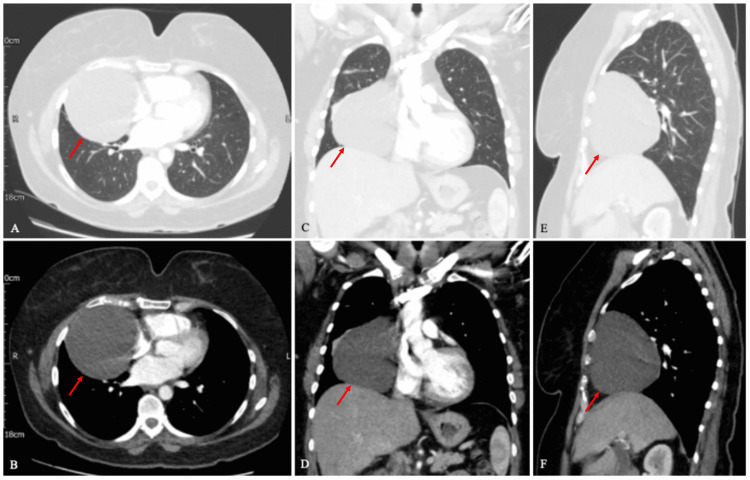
A computed tomography (CT) scan of the chest showing a large, homogenous, well-circumscribed lesion in the right lower hemithorax, and abutting the right cardiac border (red arrow). (A, C, E) Lung window (demonstrating the lung parenchyma in detail, including the pulmonary vasculature) in axial, coronal, and sagittal views, respectively. (B, D, F) Mediastinal window (demonstrating the chest wall and pleura) in axial, coronal, and sagittal views, respectively.
